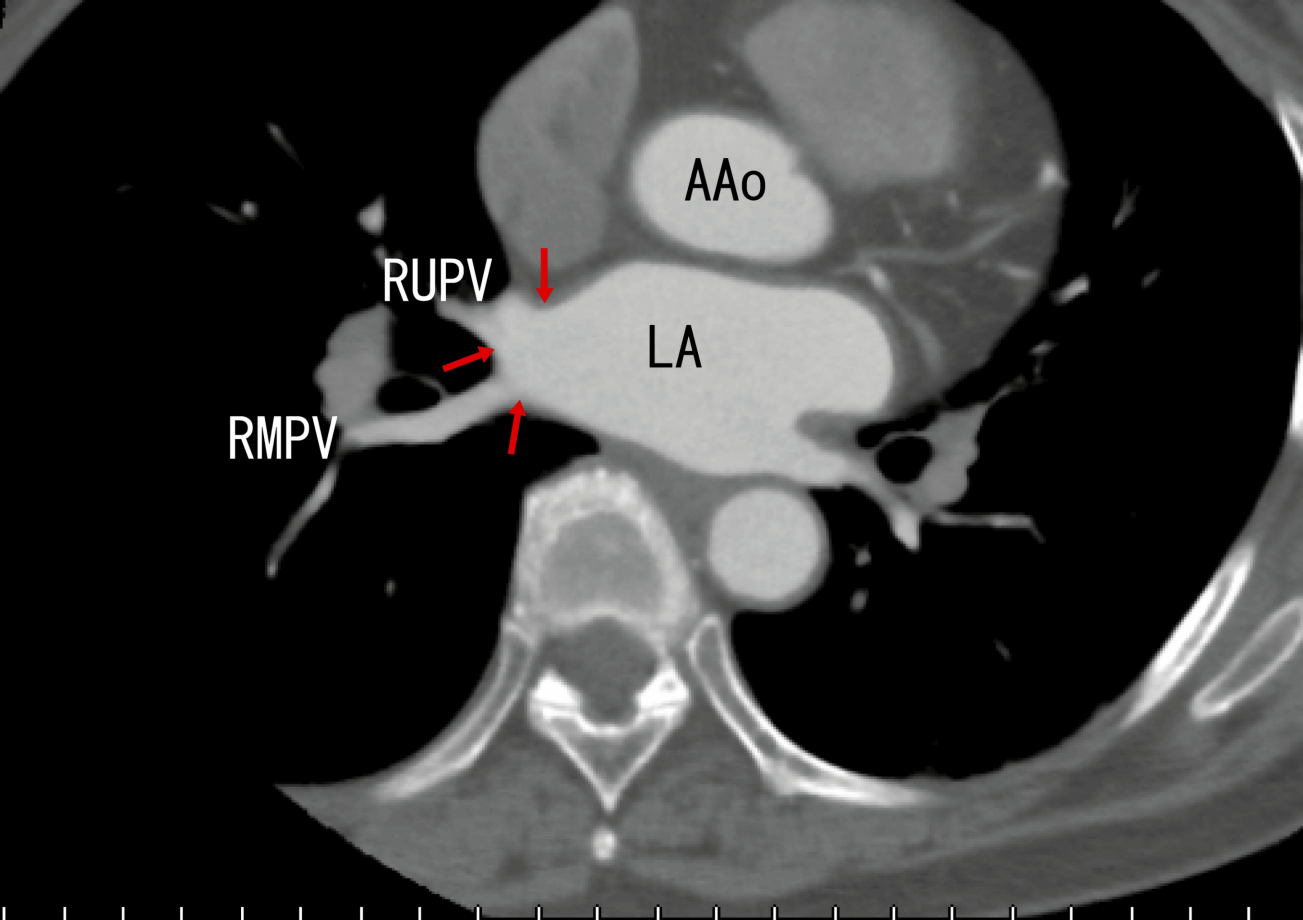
Axial enhanced CT images The axial images revealed no thrombi around the exit of the RMPV or RUPV (arrows). AAo: ascending aorta, LA: left atrium, RMPV: right middle pulmonary vein, RUPV: right upper pulmonary vein
